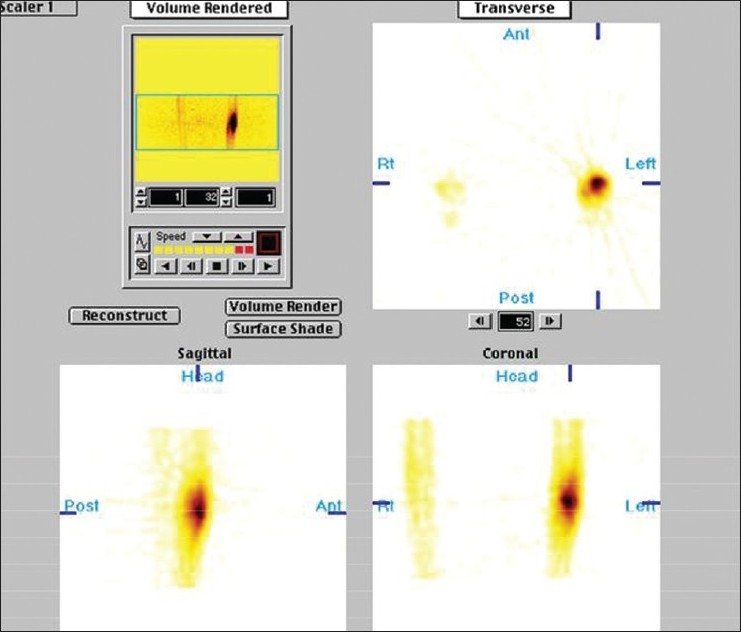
SPECT showing central active nidus with decremental activity toward the periphery, typical of an osteoid osteoma
